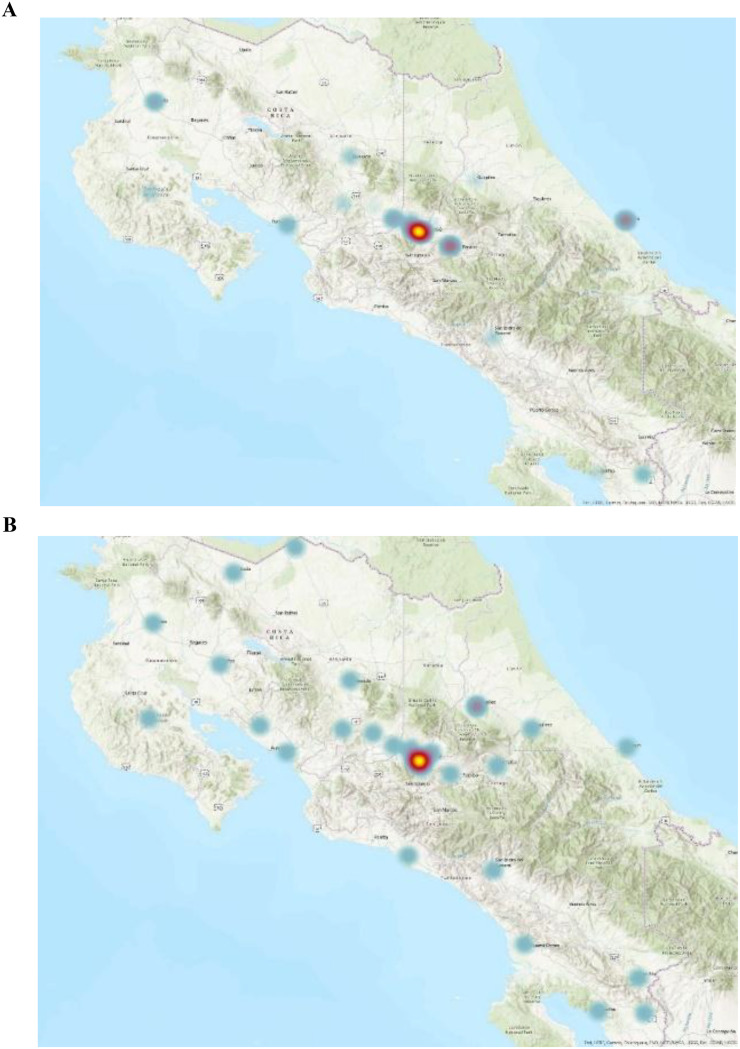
Heat map distribution of total ultrasound exam utilization (including point-of-care and consultative ultrasound) within Emergency Department and urgent care settings in 2006 and 2022. Denser utilization corresponds to warmer colors, while sparser utilization corresponds to cooler colors. New areas of ultrasound activity are represented by emergent regions of heat signal over time
